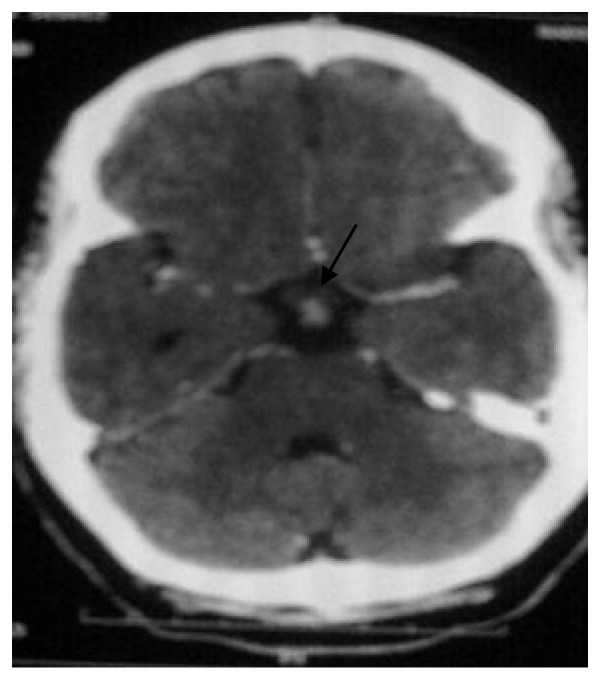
CT scan of the brain with contrast showing the suprasellar lesion.
